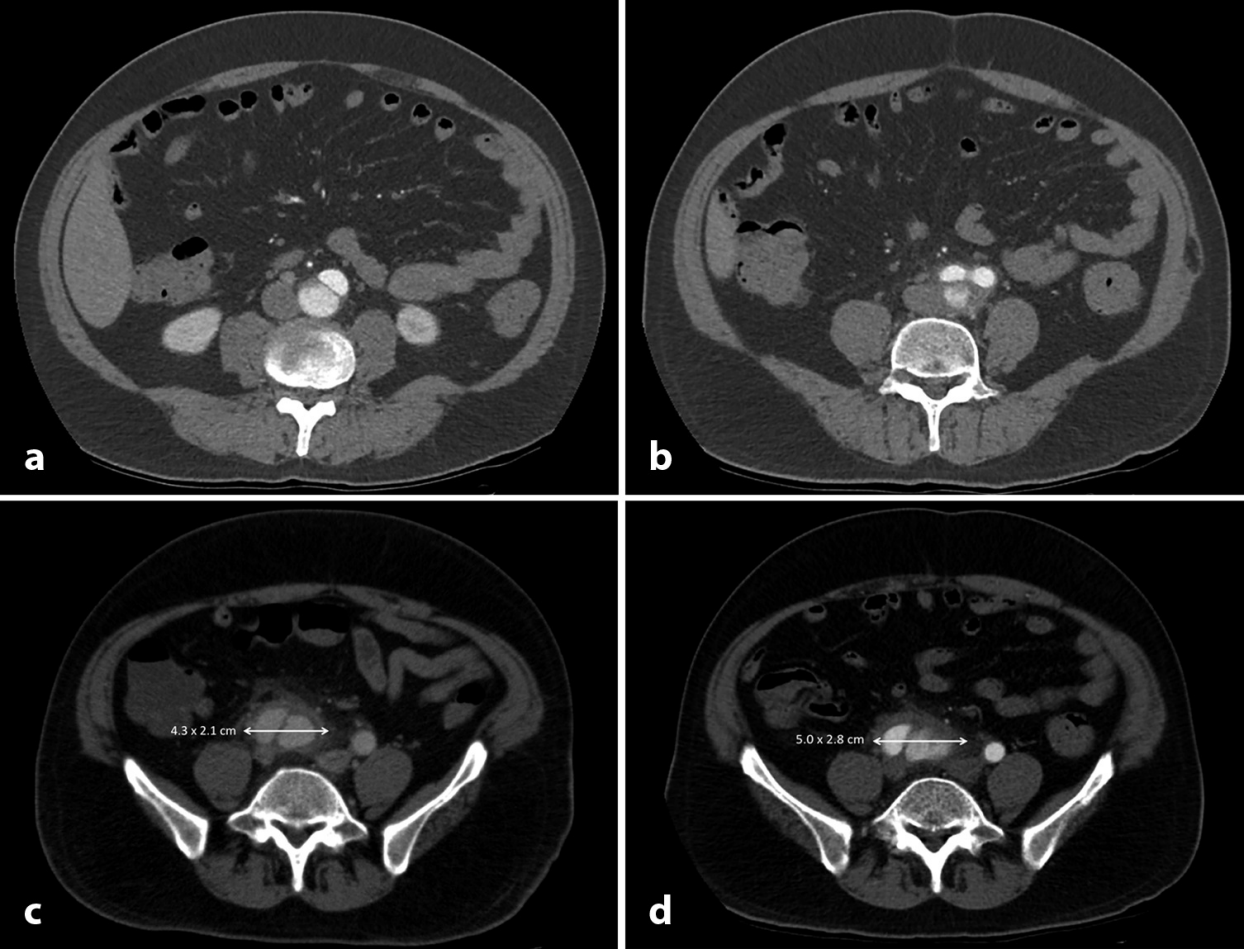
Pre-operative arterial phase (a, b and d) and venous phase (c) multidetector CT scans with 0.1 cm slices through the abdomen and pelvis. Axial images show the dissection arising in the infrarenal aorta (a) and extending across the aortic bifurcation (b) to the right internal iliac artery (c and d). Periaortic fat stranding indicates indolent infection and inflammation. Despite conservative management, persistence of inflammatory changes could be seen with an increase in the size of the right common iliac aneurysmal sac from 4.3 × 2.1 cm (c) to 5.0 × 2.8 cm (d) 6 days later. Such rapid aneurysmal dilatation seen in less than 1 week indicated that, if left untreated, the aneurysm was at risk of further expansion and rupture.
